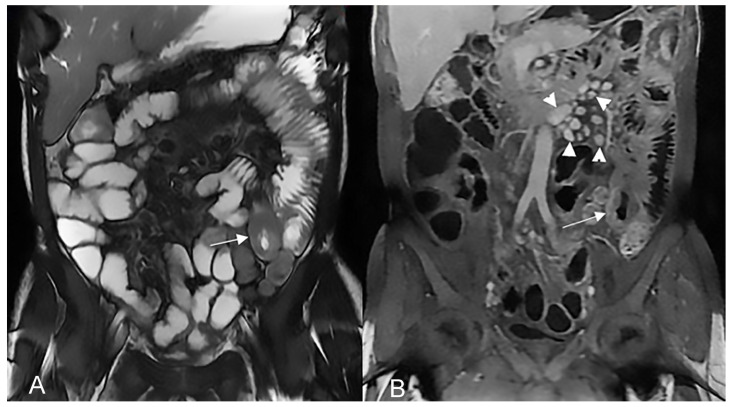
An example of eosinophilic enteritis. (A) Coronal T2-weighted fat-suppressed and (B) coronal T1-weighted after gadolinium injection images show focal thickening of a jejunal loop (arrow) with multiple enlarged lymph nodes in the mesentery (arrowheads in (B)).
